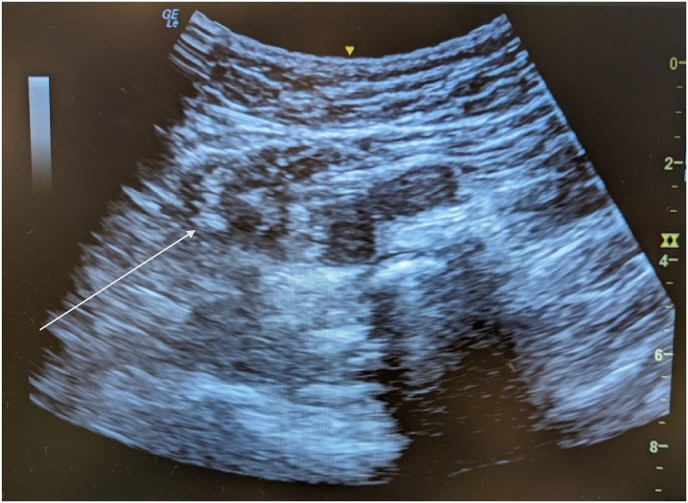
Ultrasound-guided injection. Left sciatic neurofibroma (indicated by arrow sign) visualized on ultrasound during perineural steroid injection.
